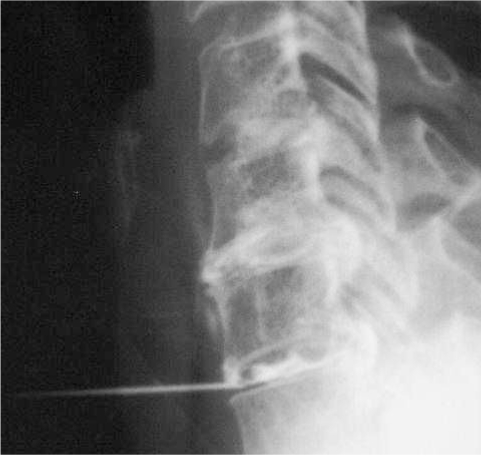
Cervical discography in a 49-year-old woman with neck pain. Lateral radiographic projection shows a normal C4/5 disc, and degenerate C5/6 and C6/7 discs with posterior protrusions. Note anterior approach used for needle placement. The needles for the upper 2 discs have been removed.
